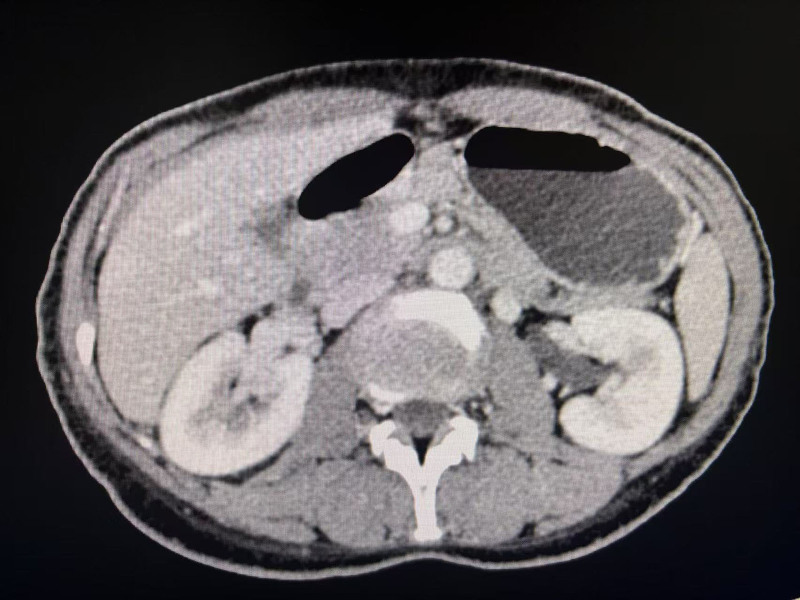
The full abdominal enhanced CT scan reveals ovarian varicosity. The asterisk denotes the presence of varicose ovarian vein.
